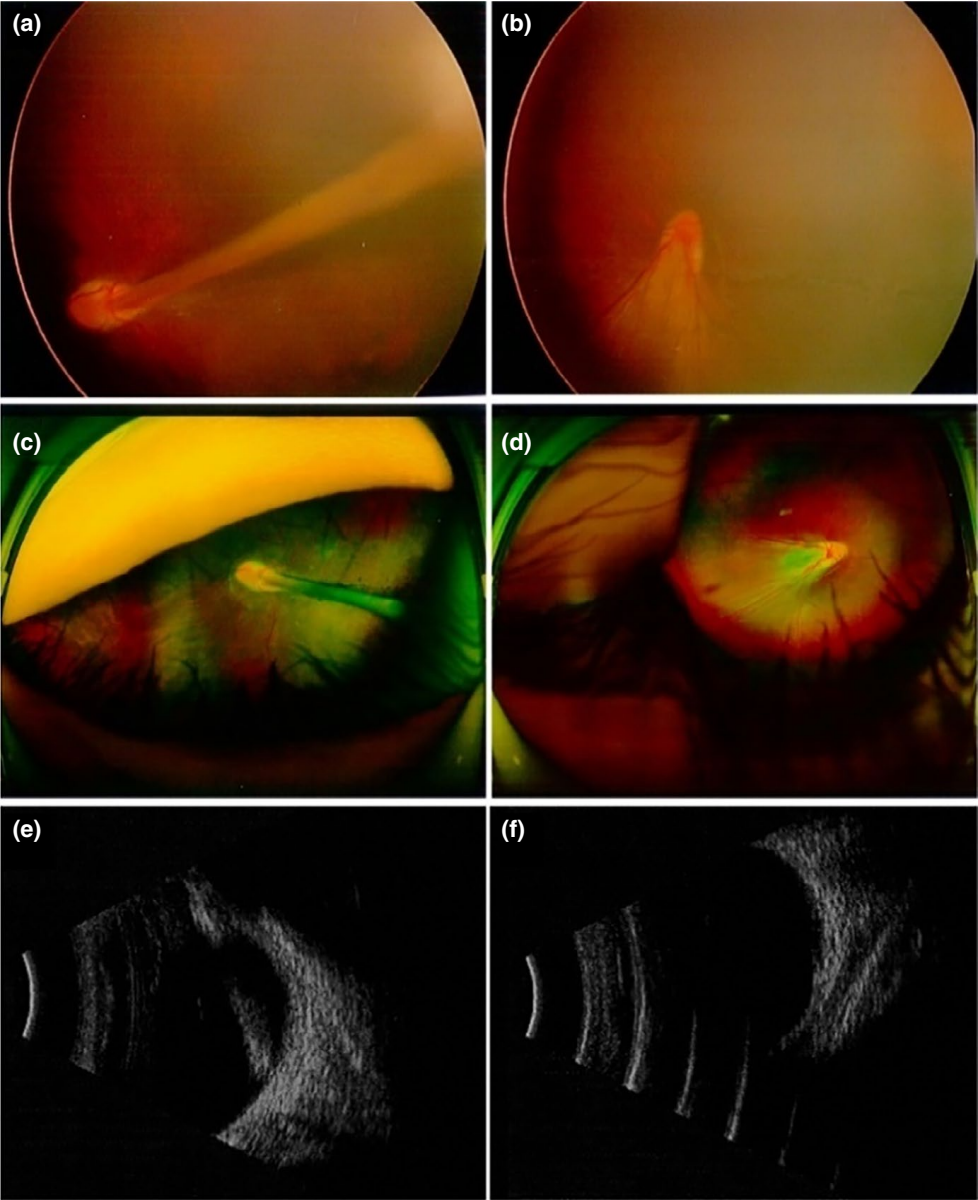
Subject IV‐1: (a, c, e) represent left eye, (b, d, f) represent right eye. B‐ultrasound, respectively, shows the scattered light spot in the vitreous cavity of the right eye, the eyeball wall of the temporal disc is not smooth, and the short light band in front of the nipple; the denser light spot in the vitreous cavity of the left eye, and the thick light echo from the nipple to the front (e, f)
